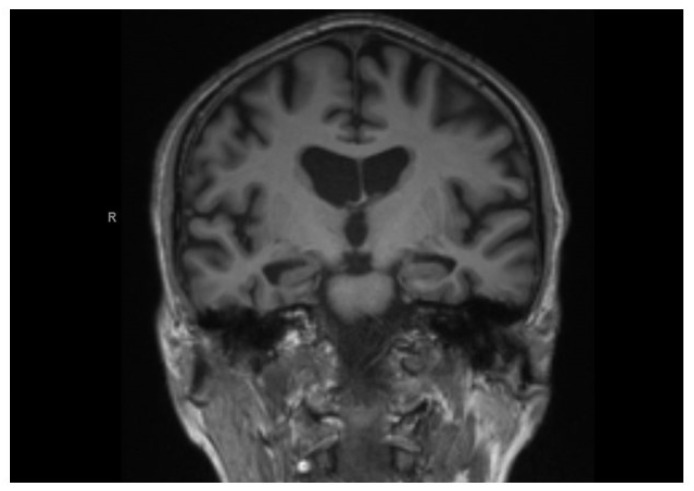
Magnetic resonance imaging picture in a patient enrolled in the study with abnormal MTA score.
